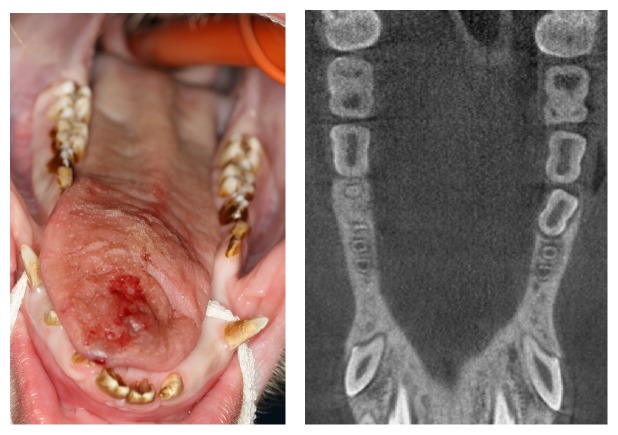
Clinical assessment and CBCT before surgery.
